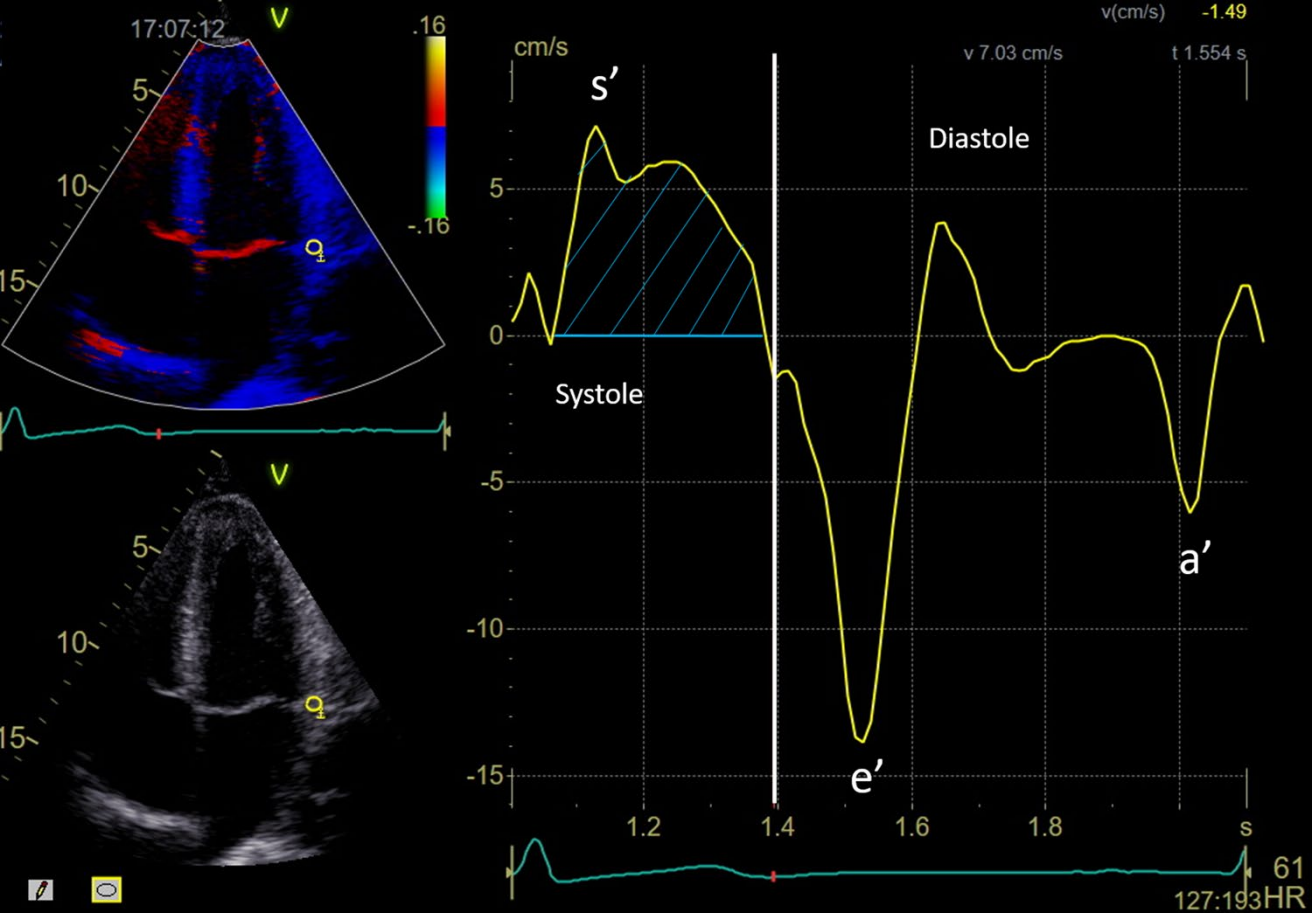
Example of myocardial velocity curve by tissue Doppler imaging. The yellow circle shows the place where the sample is obtained (lateral region of the mitral annulus). Y-axis: myocardial velocity (cm/s). X-axis: time (seconds). The positive peak during systole is marked by s’ on the velocity curve. During diastole, early (e’) and late (a’) diastolic peak is marked. Mitral annular displacement (longitudinal displacement) is calculated from area under the curve during systole (shaded area)
